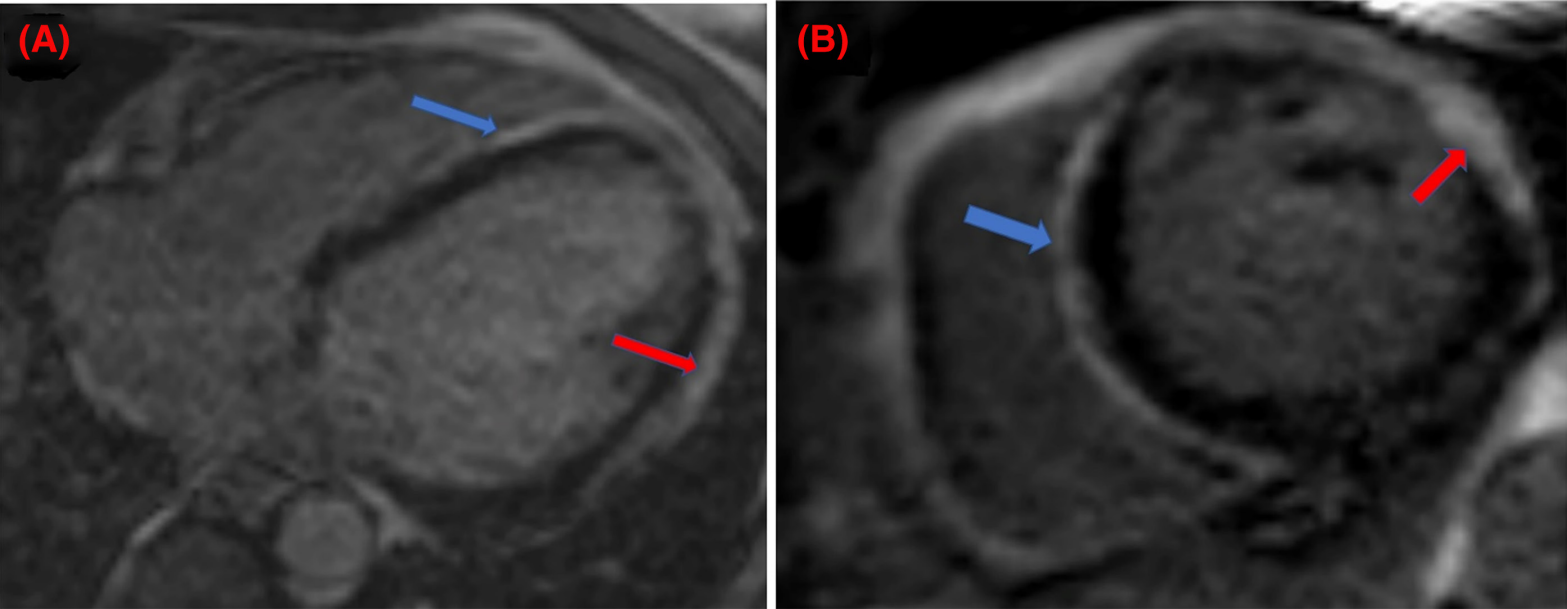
(A) Post‐contrast cardiac MRI axial view showing late‐gadolinium enhancement along the interventricular septum (blue arrow) and lateral left ventricular wall (red arrow). (B) Post‐gadolinium cardiac MRI short‐axial view showing increased signal intensity in the interventricular septum (blue arrow) and left ventricle (red arrow).
